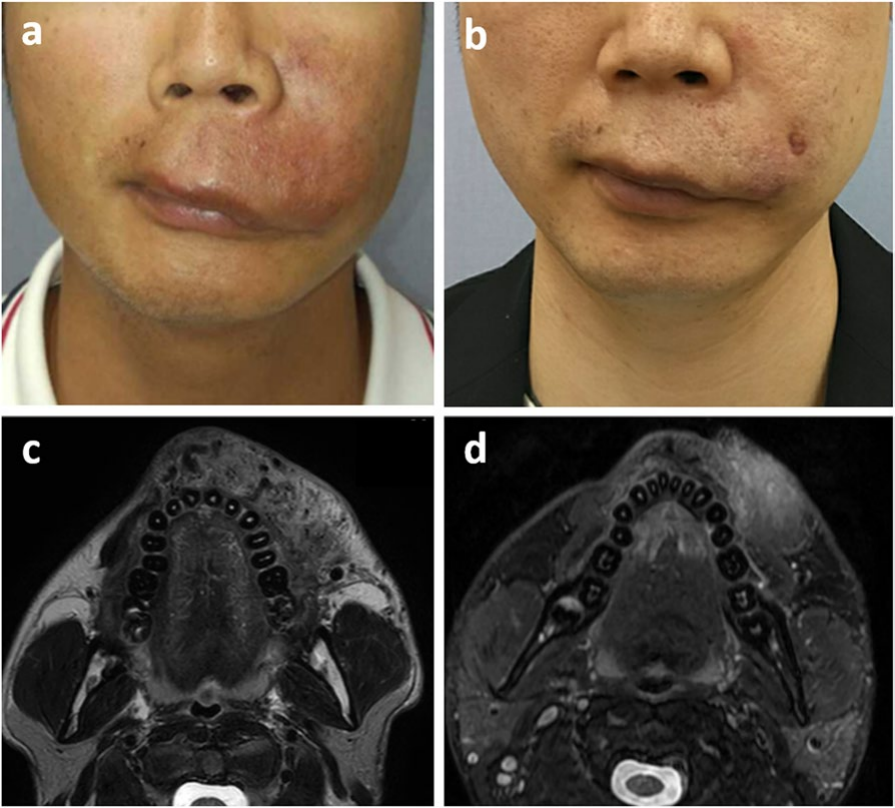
(a) Frontal view of the patient’s face in Case 1 before treatment showing swelling and erythema of the left upper lip and cheek, but no ulceration. (b) Although a small erosion occurred about a week after treatment then a scar remained on the left upper lip, swelling and erythema of the lip and cheek had resolved 6 months after the fifth embolization session. (c) T2WI MRI before treatment showing hyperintensity of the soft tissues, which were permeated by massive flow voids representing enlarged feeding and draining vessels. (d) Six months after the final session, fat-suppressed T2WI showing regression of the soft tissue swelling and disappearance of the flow voids
